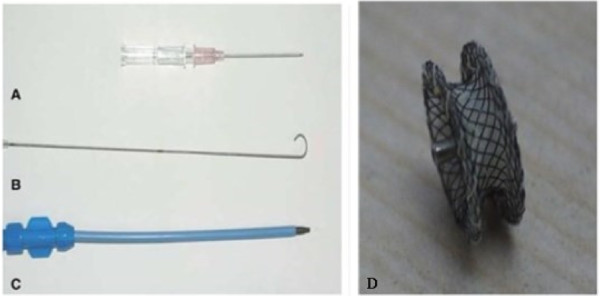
There were transthoracic minimally invasive closure devices. A was the puncture needle; B was the guide wire with a soft and floppy end; C was the livery sheath; D was the vsd occluder.
